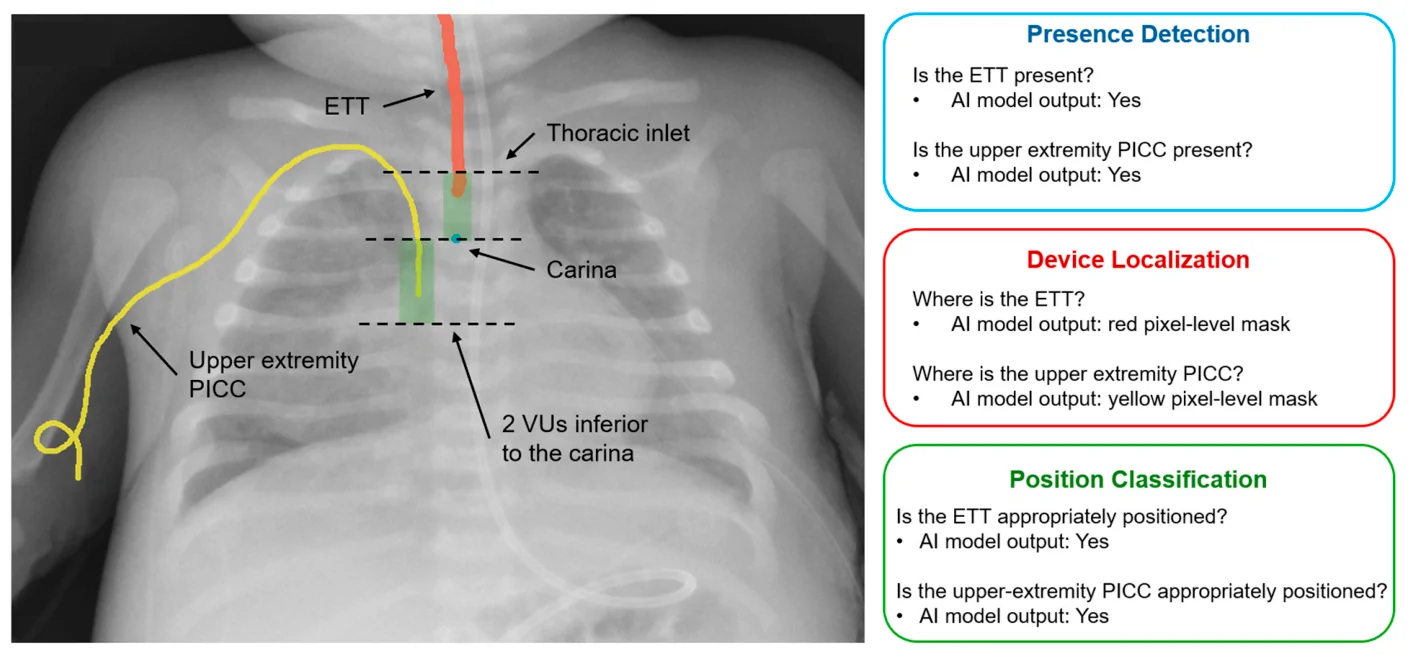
Conceptual overview of three AI tasks applied to LDA assessment on chest radiography. Representative neonatal chest radiograph demonstrating three common AI paradigms for LDA assessment: (1) Presence Detection: determining whether a targeted LDA is present (e.g., ETT present: yes; upper extremity PICC present: yes). Untargeted devices present on the radiograph (such as the enteric tube and chest tube) are not evaluated by the AI models. (2) Device Localization: segmenting the targeted LDA full course and/or identifying its distal tip (e.g., red pixel-level mask for ETT, yellow mask for upper extremity PICC). (3) Position Classification: determining whether the LDA distal tip is appropriately positioned relative to anatomical landmarks (e.g., Is the ETT appropriately positioned? Yes. Is the upper-extremity PICC appropriately positioned? Yes). ETT, endotracheal tube; LDAs, lines, drains, and airways; PICC, peripherally inserted central catheter; VU, vertebral unit.
